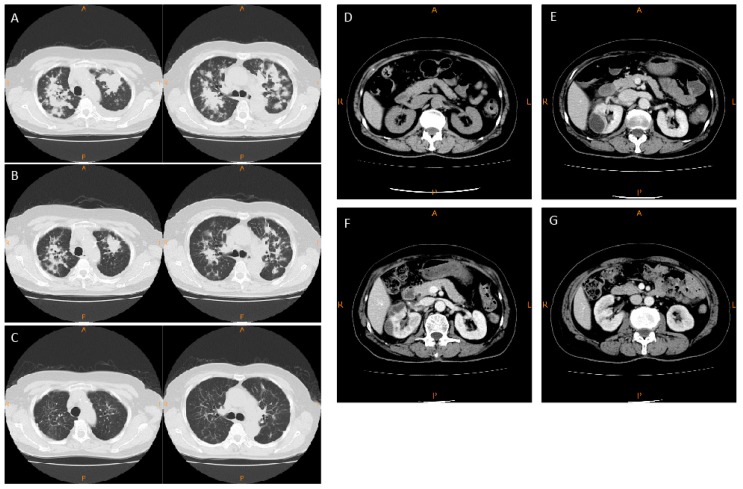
Chest and abdominal computed tomography (CT) in the present case. Chest CT of the patient with *ALK*-positive NSCLC at diagnosis (A), after 1 month (B), and after 11 months (C) of crizotinib therapy. Abdominal CT of the patient before therapy with crizotinib (D), 11 months after crizotinib therapy (E), 13 months (F) after crizotinib therapy, and after stopping the drug (G). NSCLC: non–small cell lung cancer; *ALK*: anaplastic lymphoma kinase.
